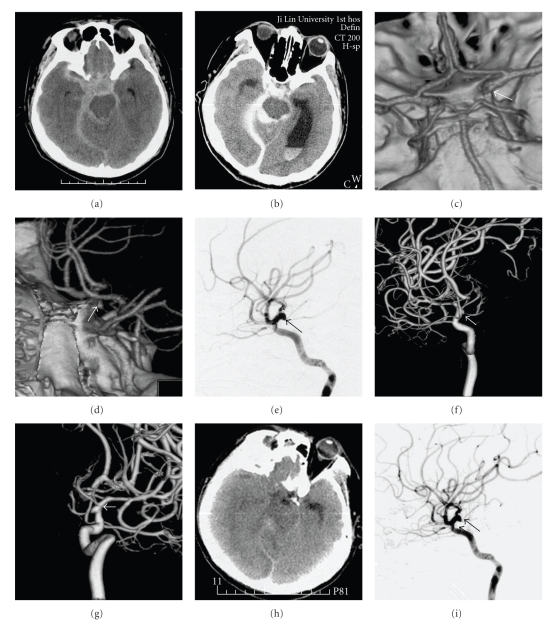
Diagnosis of ruptured ID of the PCo-A. (a, b) Two SAH images detected at an interval of 9 hours show that the bleeding points in both episodes of hemorrhage were centered around the origin of the right posterior communicating artery and that the suprasellar cistern, sylvian cistern, ambient cistern, and tentorial margin were involved. Blood entry into the ventricles and the presence of ventriculomegaly were seen in (b). (c, d) Head CTA showed that a funnel-shaped expansion with a diameter of less than 3 mm occurred at the origin of the right posterior communicating artery and was in conjunction with the thin right posterior communicating artery at its apex (arrow). (e)–(g) The first DSA showed that a funnel-shaped expansion occurred at the origin of the right posterior artery and was in conjunction with the thin right posterior communicating artery at its apex, a finding similar to the results of CTA (arrow). (h) Reexamination by head CT after 20 days of conservative treatment demonstrated that SAH was absorbed and the size of ventricles was normal. (i) The second DSA examination after 20 days of conservative treatment revealed that the funnel-shaped expansion still existed at the origin of the right posterior communicating artery and was in conjunction with the thin posterior communicating artery at its apex, similar to the results obtained by CTA and the first DSA (arrow).
